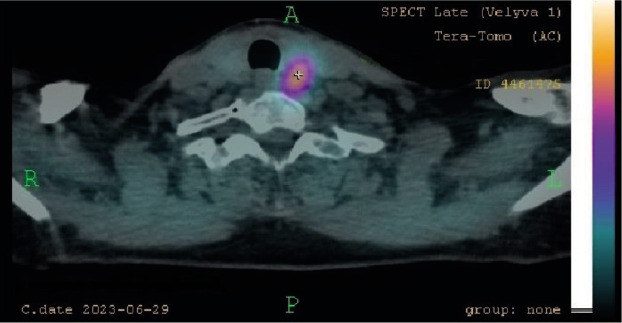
SPECT, late phase. Persistent retention of the radiotracer.
